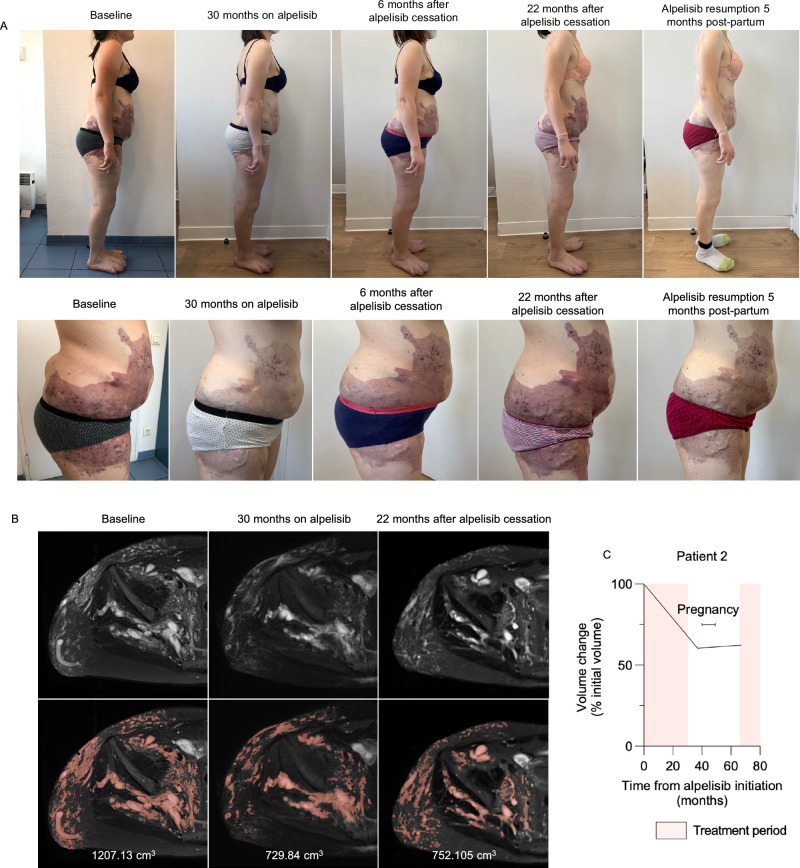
Patient 2 following alpelisib withdrawal and reintroduction. A Representative pictures of patient 2 over time. B Representative transversal MRI T2 fat sat sequences of patient 2 over time. C Magnetic resonance volumetric of the malformation over time.
